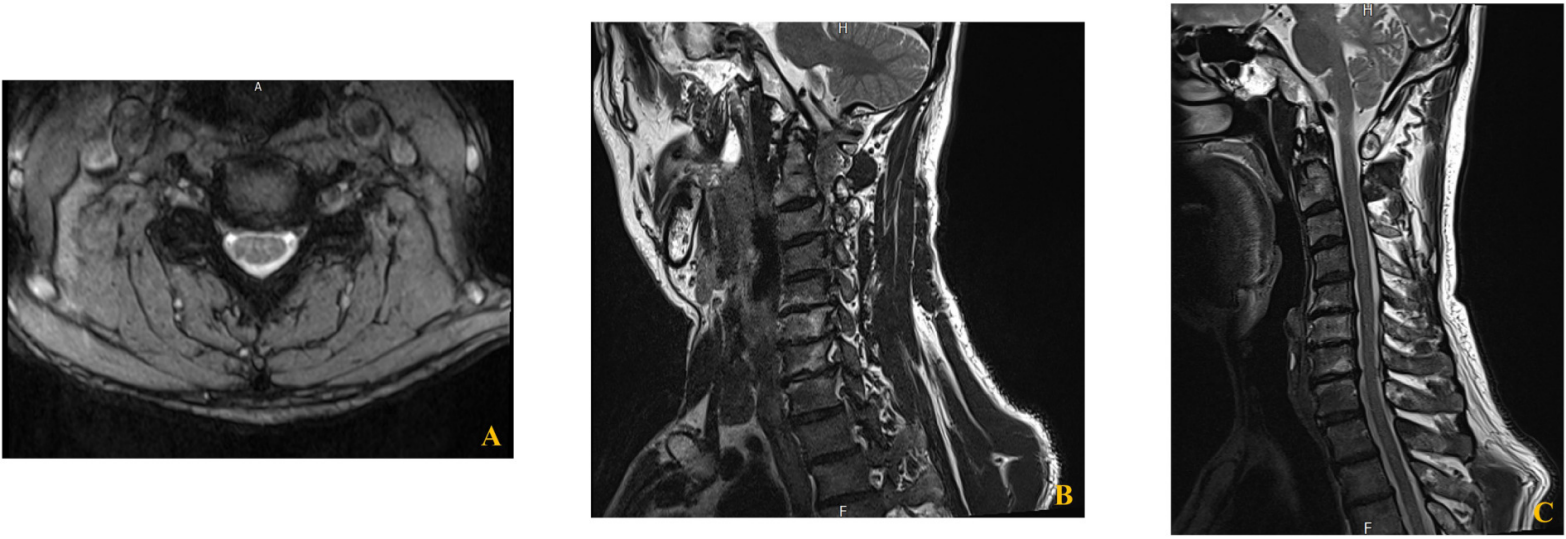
Cervical spine MRI demonstrating right sided foraminal stenosis from C5 to T1. (A) Axial view showing cervical spinal canal and neural foraminal anatomy. (B) Right oblique view with visible cervical vertebrae (C4–C7) demonstrating right-sided foraminal stenosis. (C) Sagittal view showing the cervical spine alignment and neural foraminal narrowing.
